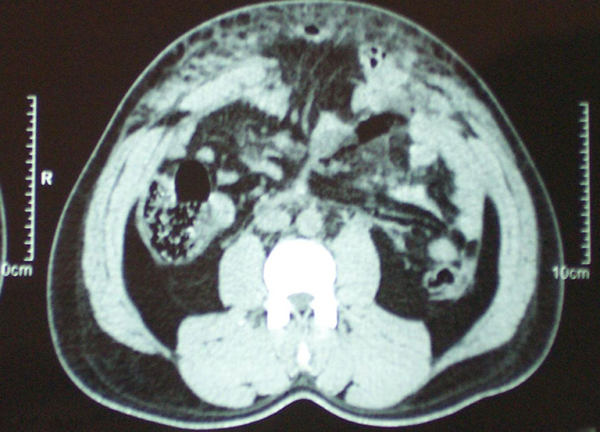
Contrast enhanced computed tomography showing traumatic hernia in the same patient. Supra-umbilical defect in the midline anterior abdominal wall (maximum diameter 5 cm), with herniation of small bowel loops and mesentery through it, with extensive interstitial edema.
